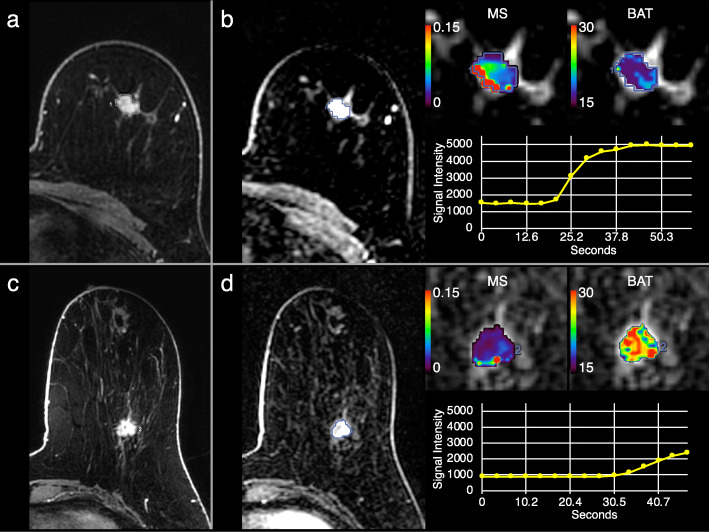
Dynamic contrast-enhanced magnetic resonance images (DCE-MRI) and parametric maps of representative cases. a, b Triple negative type invasive ductal carcinoma (IDC) in the left breast of a 55-year-old woman. c, d Luminal type IDC in left breast of a 54-year-old woman. Standard DCE-MRI (first post-contrast phase; a, c) and ultrafast DCE-MRI (15th phase, generated parametric maps, and signal intensity curves; b, d) are shown. The triple negative type IDC showed rapid increase of signal intensity with maximum slope (MS) of 0.145 mmol/s and bolus arrival time (BAT) of 18.8 s. The luminal type IDC showed slow increase of signal intensity with MS of 0.054 mmol/s and BAT of 27.7 s
